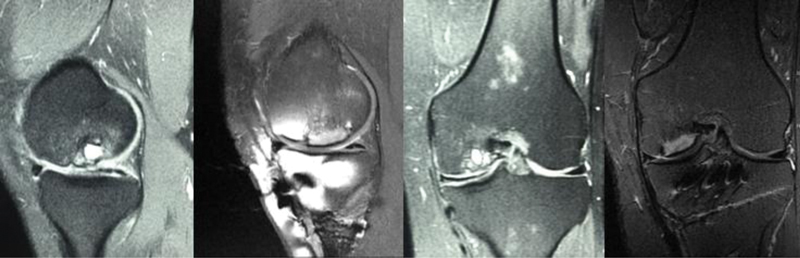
Coronal and sagittal magnetic resonance imaging sequences showing allograft integration one year postoperatively. The metal artifact consists of the fixation material of the osteotomy.
